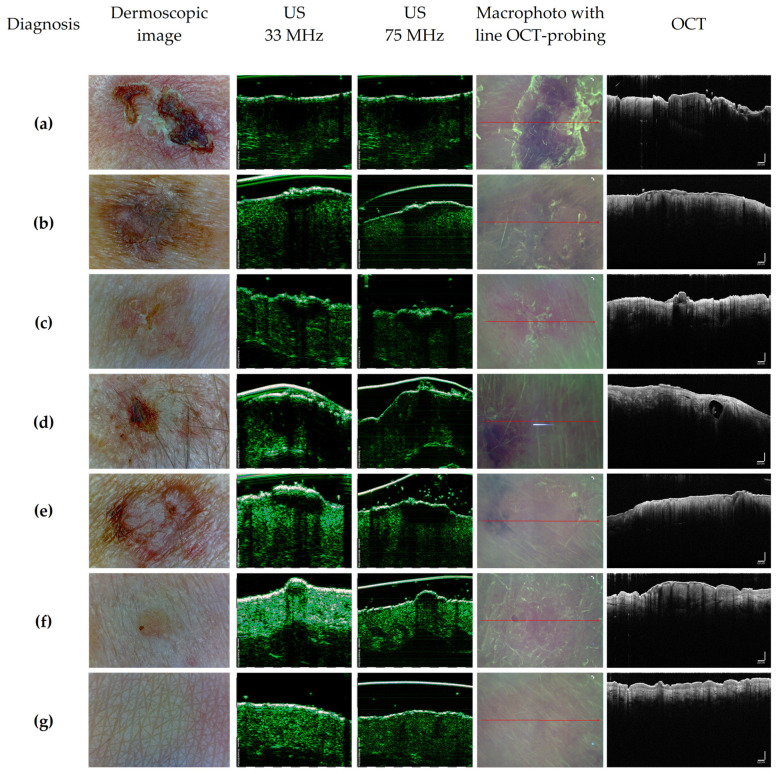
Typical dermoscopic images, ultrasound images at a central frequency of 33 MHz and 75 MHz, macro images with the plane and direction of OCT scanning marked with a red arrow and OCT scans of skin neoplasms of volunteers with a diagnosis of BCC infiltrative–ulcerative (a), pigmented (b), superficial (c), morpheaform (d), nodular (e), benign neoplasm (f) and visually healthy skin (g). The height and width of ultrasound images were 3.2 and 13 mm, and those of OCT images were 2.7 and 6 mm.
